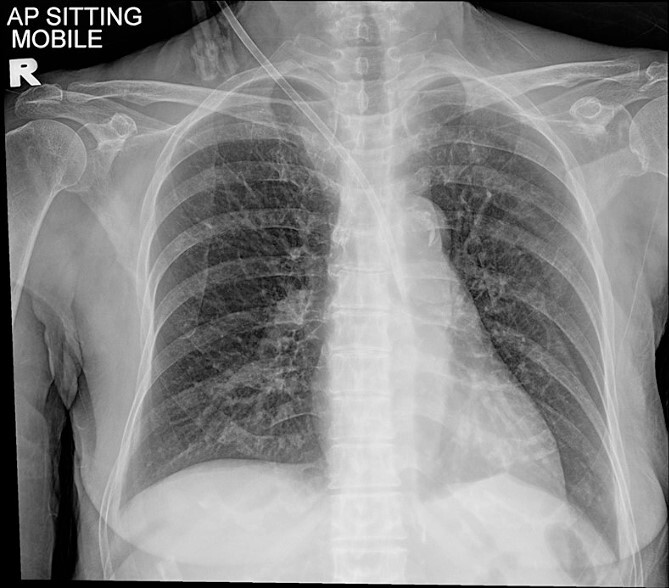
Chest radiograph showing a malpositioned central vein catheter.
